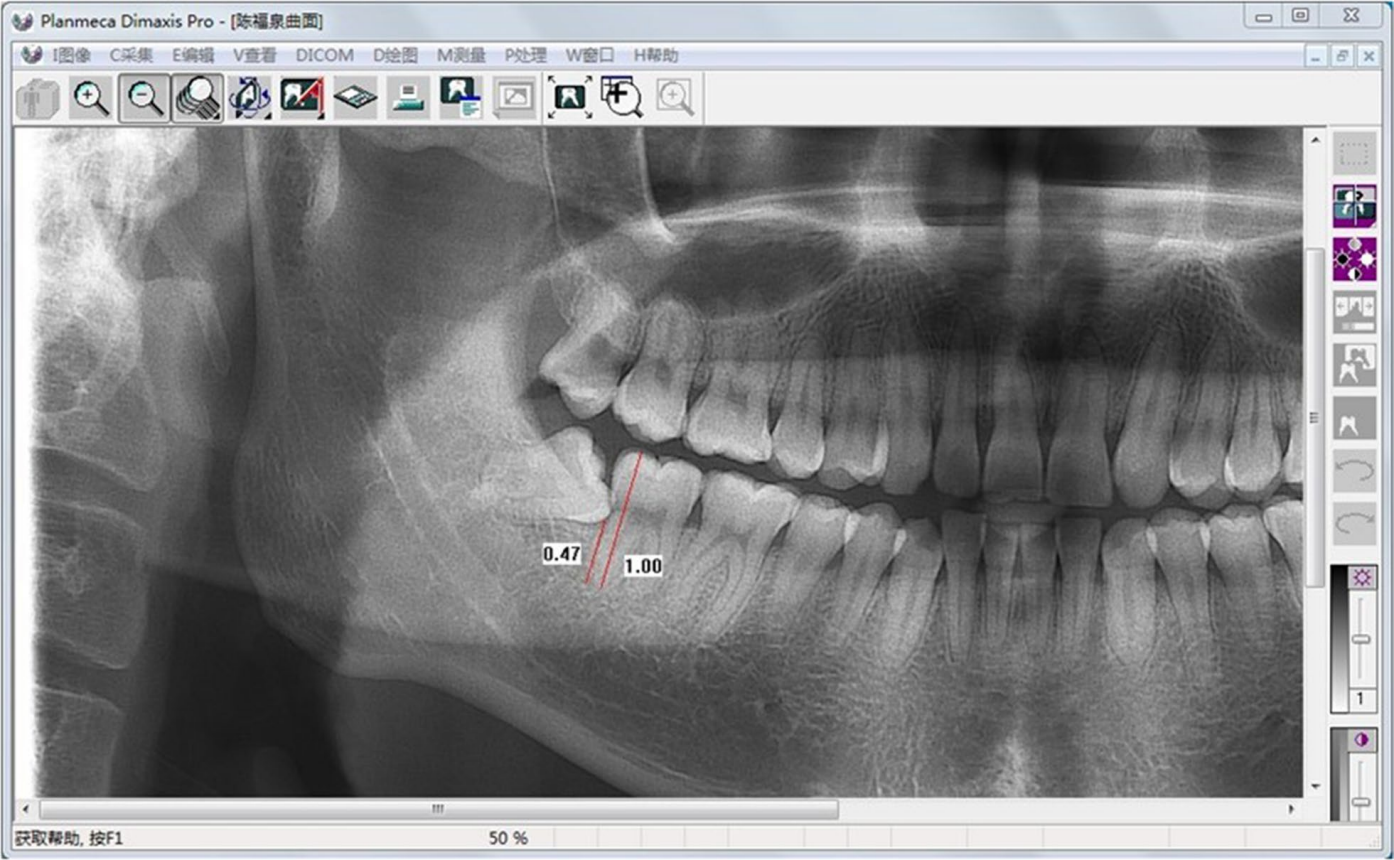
Perioperative radiograph patient shows the site for measuring bone height
